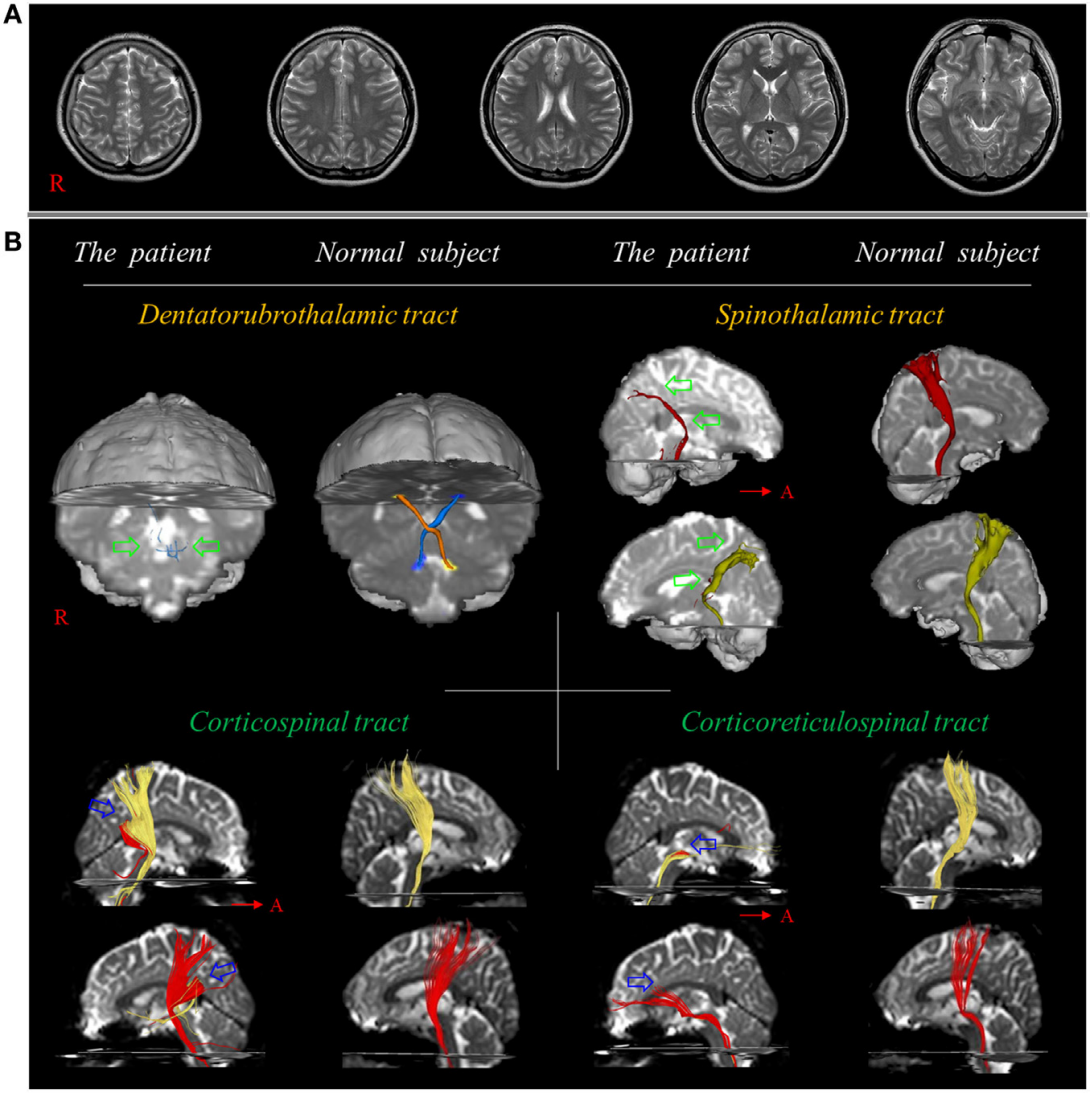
(A) T2-weighted brain MR images at 10 weeks after onset show no abnormality. (B) Results of diffusion tensor tractography. Only tiny fibers (green arrows) of the dentato-rubro-thalamic tract are reconstructed at the brainstem level and the spinothalamic tract is thinner in both hemispheres (green arrows) compared with a normal subject (26-year-old female). By contrast, the corticospinal tract and corticoreticulospinal tract show partial tearing and discontinuation at the subcortical white matter level in both hemispheres (blue arrows). Reprinted from Jang and Lee (26) with permission.
